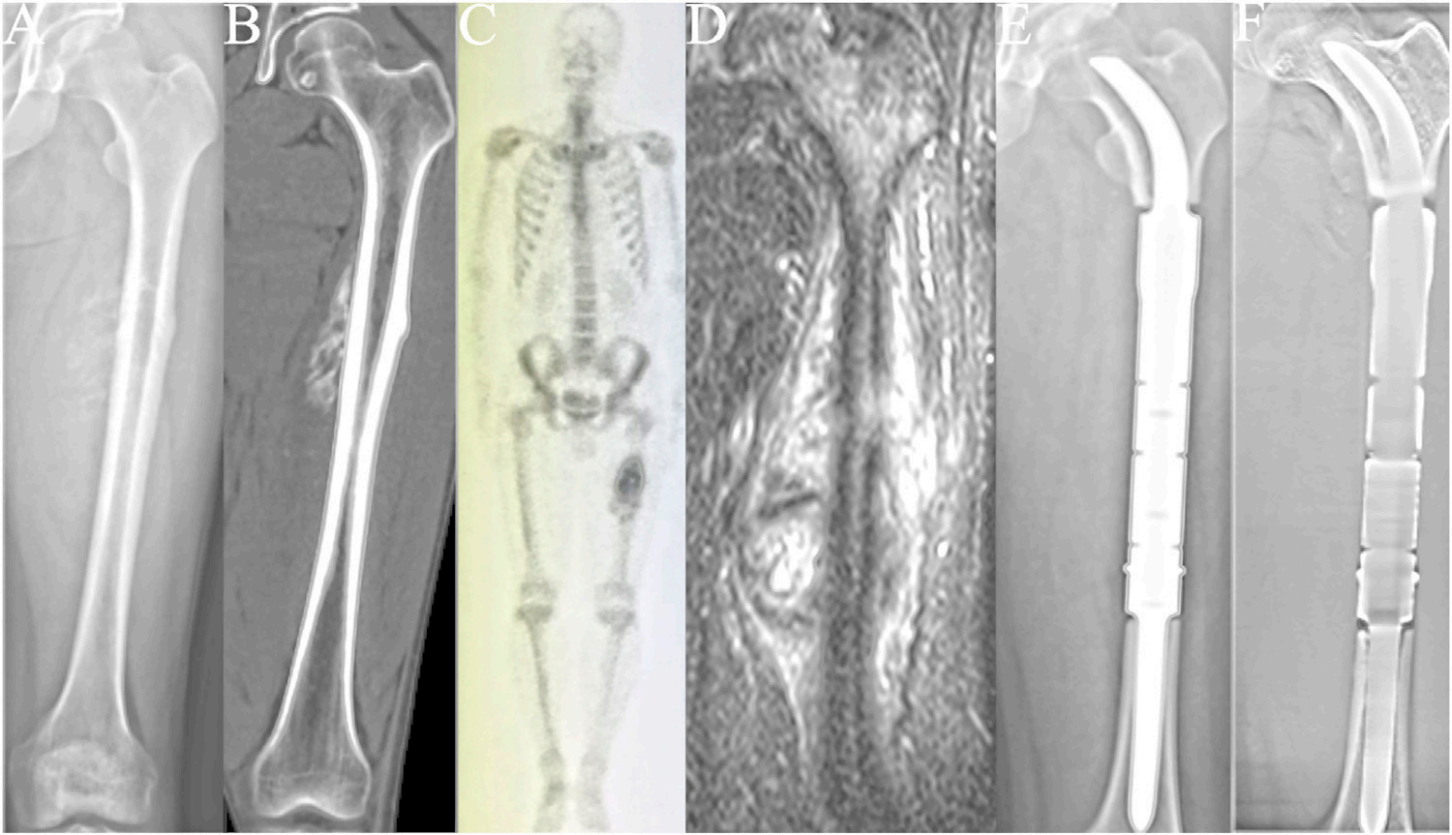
All patients underwent *en bloc* tumor resection followed by reconstruction with the customized cementless intercalary endoprosthesis with an intra-neck curved stem. (A) Anteroposterior radiograph of the left femur of a patient with a femoral diaphyseal osteosarcoma. (B) Computed tomography (CT) image of the left femur. (C) Single-photon emission whole-body CT image. (D) Magnetic resonance image of the patient’s left upper leg. (E) Postoperative radiograph of the femur. (
